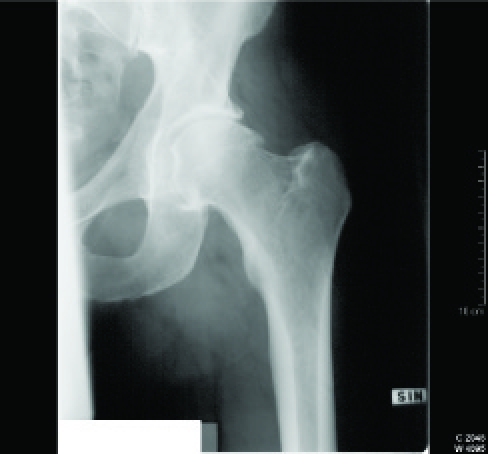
Plain radiography of the left hip.
